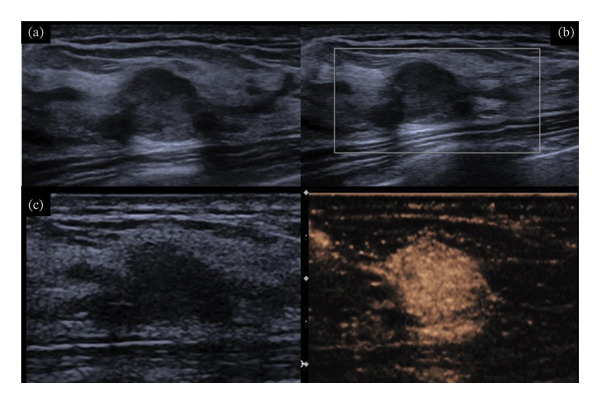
A 47‐year‐old lady with a BI‐RADS 4A lesion in the right breast. The patient reported a possible palpable mass. The maximal diameter of the lesion was 13 mm. In combination with contrast‐enhanced ultrasound, the lesion was downgraded to BI‐RADS 3. Ultrasound‐guided vacuum‐assisted excision was performed. The pathology was intraductal papilloma. (a) B‐mode ultrasound showed a hypoechoic lesion with duct change and posterior enhancement, (b) color Doppler detected no flow signal in or around the lesion, and (c) CEUS showed hyperenhancement with regular shape and similar scope.
